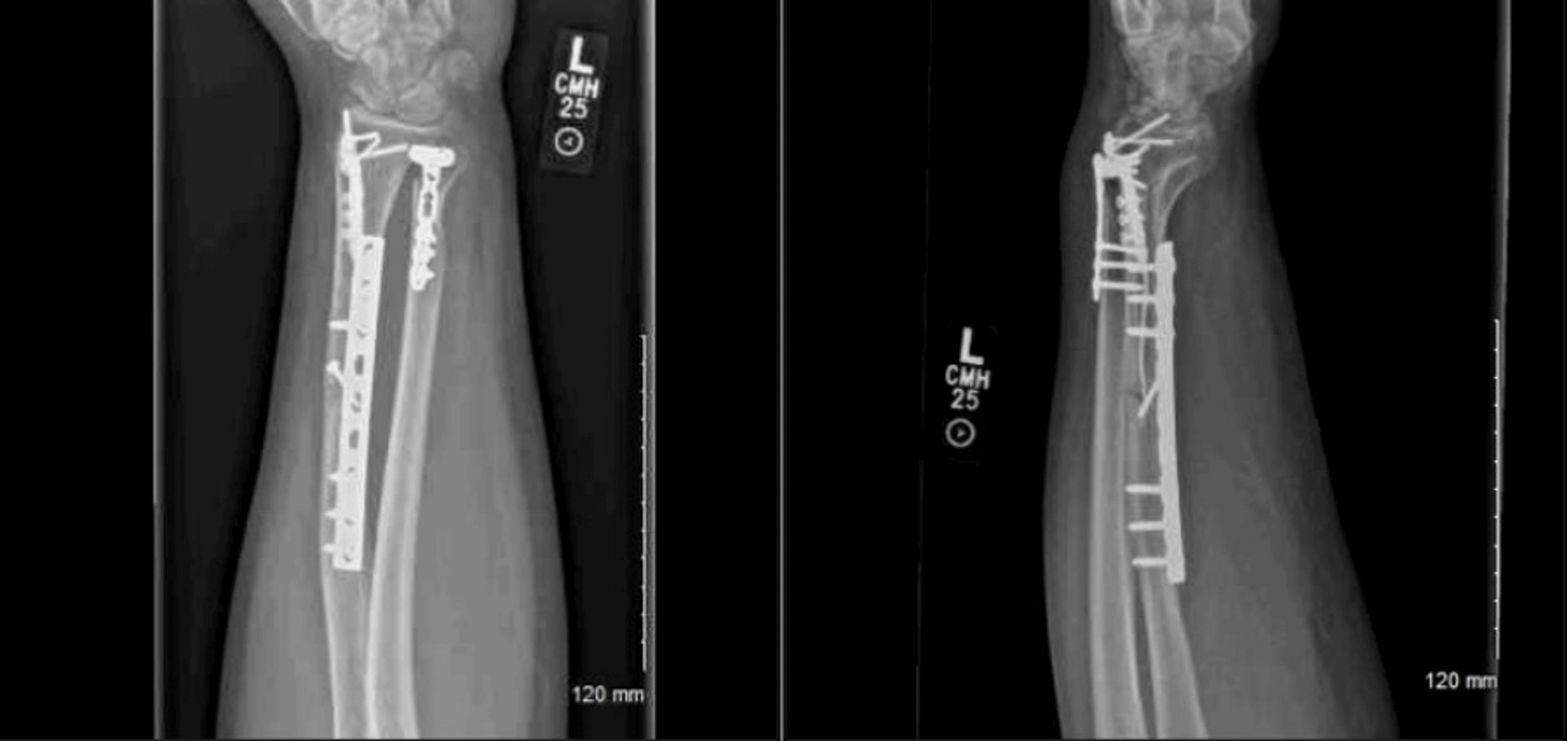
Three-month postoperative anteroposterior (A) and lateral (B) X-ray films demonstrating intact hardware and bone callus formation at the fracture sites
